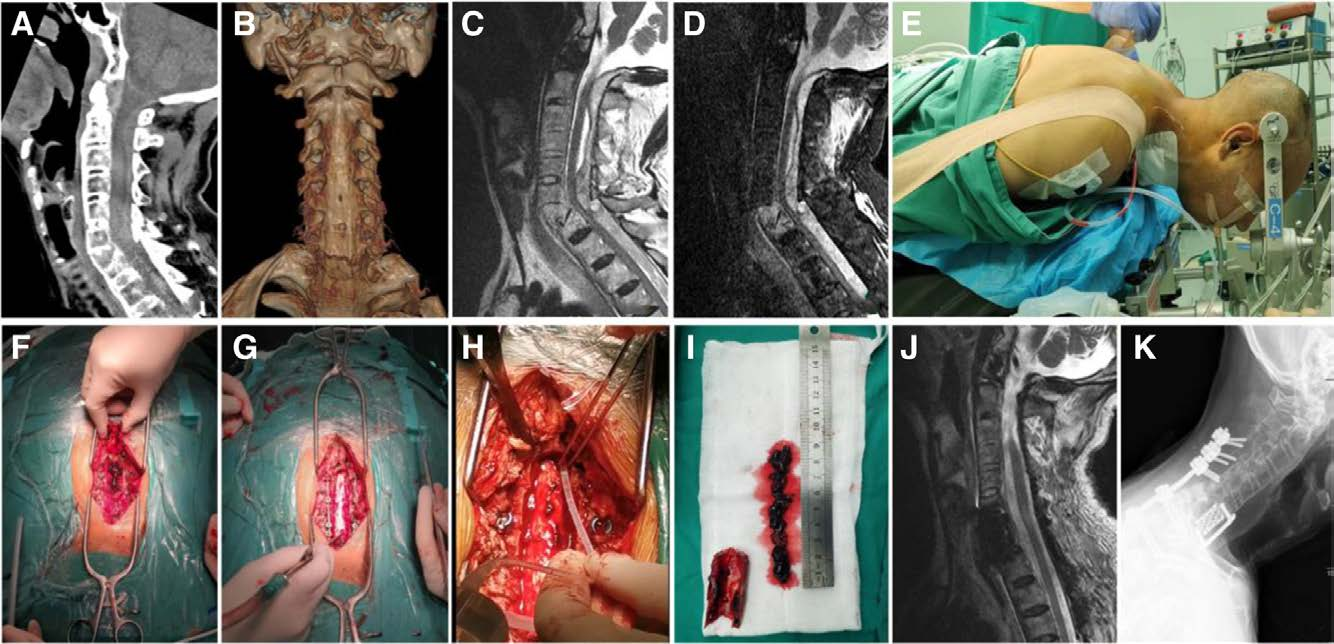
(A–B) Preoperative cervical CT scans: (A) Sagittal reconstruction demonstrates a fracture through the C7 vertebral body with associated hyperextension deformity and loss of normal cervical lordosis. Note the extensive syndesmophyte formation and ankylosis of facet joints, characteristic of advanced ankylosing spondylitis. (B) Axial image reveals comminuted fractures of the anterior elements at C7; C–D, Preoperative MRI: (C) Sagittal T2-weighted image shows a extensive posterior epidural hematoma extending from the mid-cervical to the upper thoracic spine, causing severe compression and displacement of the spinal cord. (D) Axial T2-weighted image at the C6/7 level confirms the significant cord compression and signal changes within the cord suggestive of contusion and edema; (E) Intraoperative positioning: The head was stabilized using a Mayfield head frame to ensure precise alignment and stability during the posterior procedure; (F) Intraoperative exposure: After laminectomy, dark red clots are seen covering the dorsal surface of the dura mater; (G) Decompressed spinal cord: Following hematoma evacuation, the spinal cord shows restored pulsation and adequate decompression; (H) Irrigation technique: A customized dural soft catheter connected to a syringe is being used to gently irrigate and aspirate hematoma from the spinal canal; (I) Evacuated hematoma: The aspirated clot, measuring approximately 9 cm in length; (J) Postoperative MRI: Sagittal T2-weighted image obtained prior to discharge confirms complete evacuation of the epidural hematoma and adequate decompression of the spinal canal; (k) 6-month follow-up X-ray: Lateral cervical spine radiograph demonstrates maintained alignment and stable positioning of the anterior titanium mesh cage and posterior instrumentation without signs of loosening or migration.
